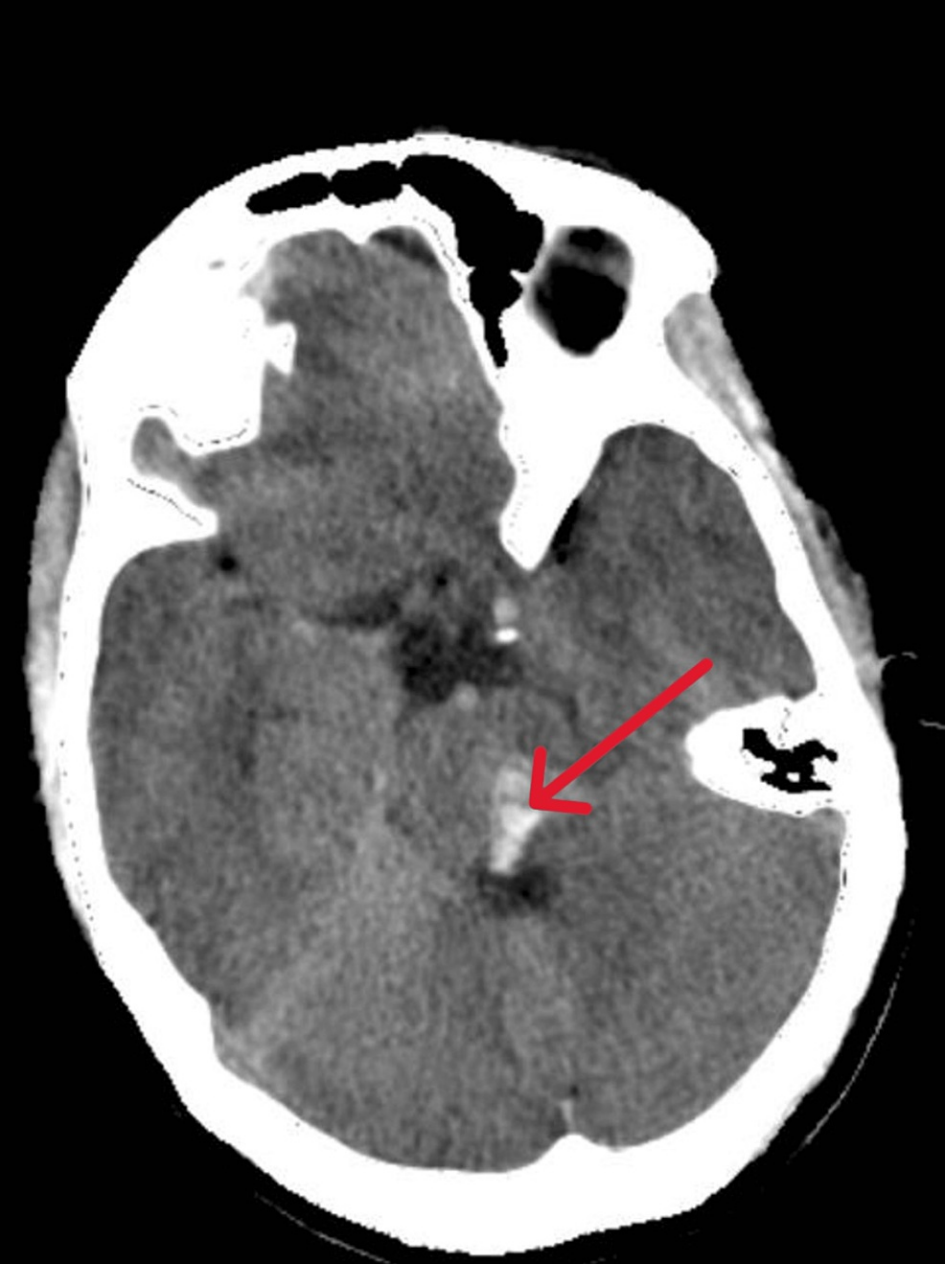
Non-contrast CT brain showing bleed in the pons Arrow points to the bleed.
